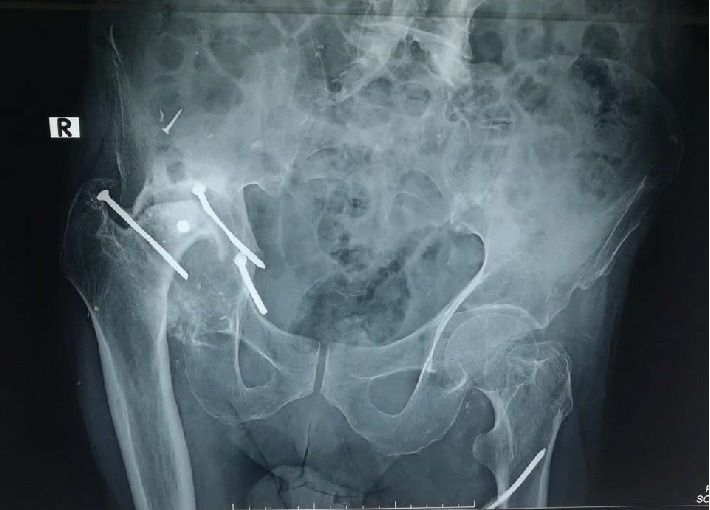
X-rays of the pelvis and both hip joint anteroposterior view showing grade 4 osteoarthritis of the right hip joint with destruction of the right femoral head due to retained shrapnel.
